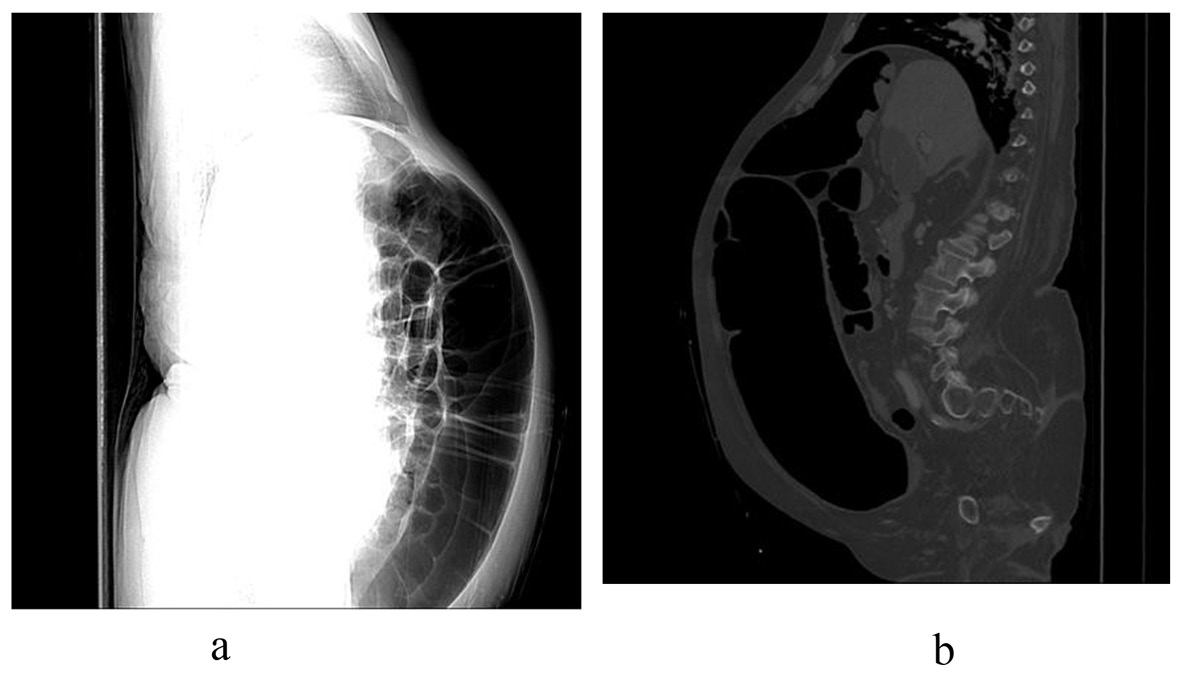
Spiral CT-Abdomen, lateral view demonstrating massive colonic dilatation.
